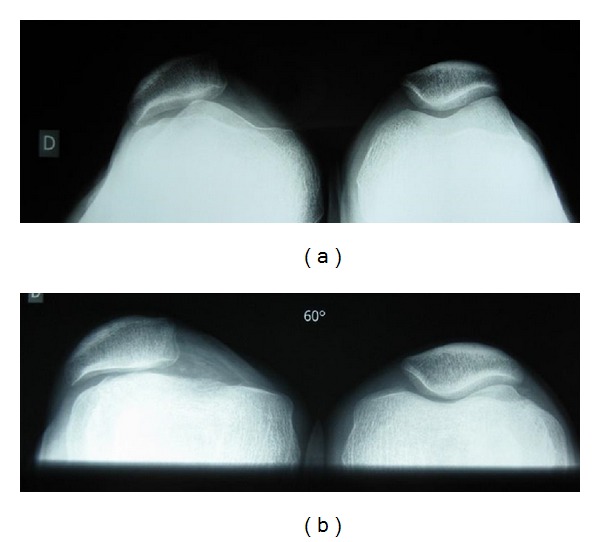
Preoperative patellofemoral X-ray, tangential view in neutral rotation: (a) 30° flexion and (b) 60° flexion.
